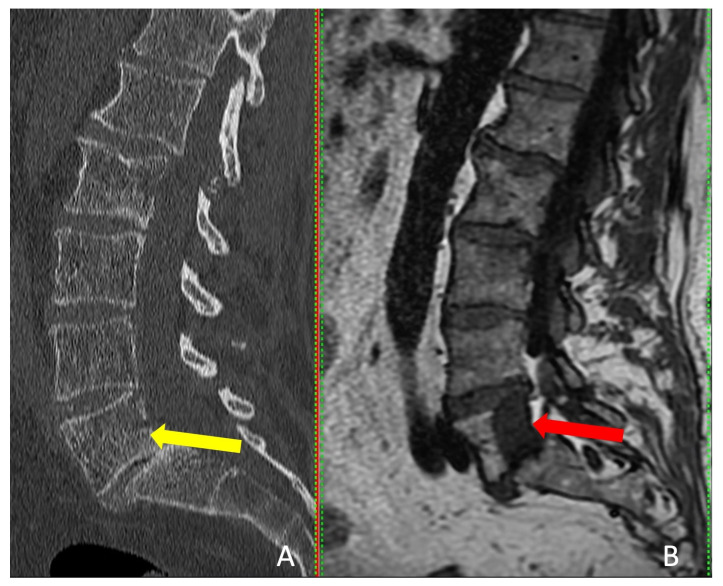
Sagittal view of CT scan (A) and MRI scan (B). The red arrow indicates the tumor and the yellow arrow shows where the tumor should be detectable.
